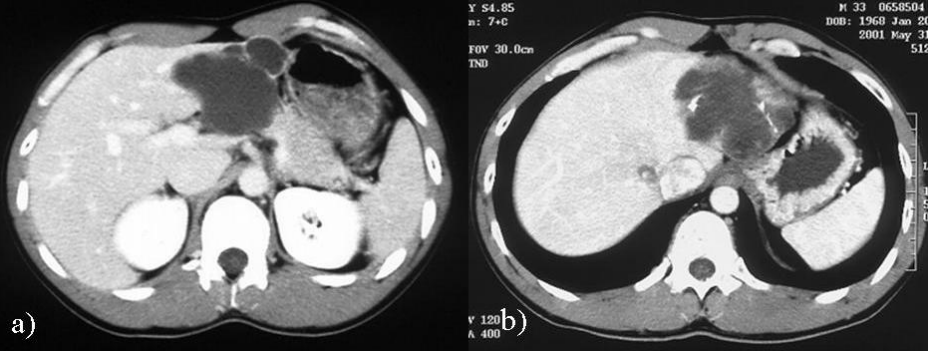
Abdominal CT findings. a) in 1996, showing the unilocular cyst 6 cm in diameter without calcification, septa and papillary projections. No contrast enhancement was seen. b) in 2001, showing the same diameter with calcification and septa.
